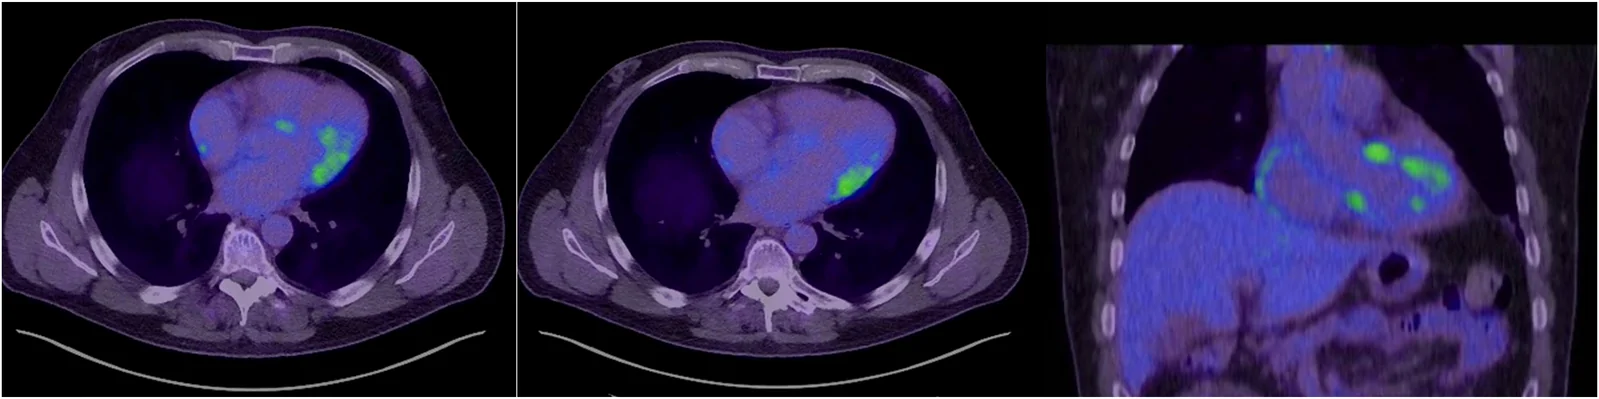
FDG-PET/CT Demonstrating Increased Myocardial Uptake Fluorodeoxyglucose positron emission tomography/computed tomography (FDG-PET/CT) demonstrates that axial cross-sectional views (left and middle panels) show uptake in the basal to mid-lateral and anterolateral segments. Sagittal view (right panel) demonstrates uptake in the basal septum.
